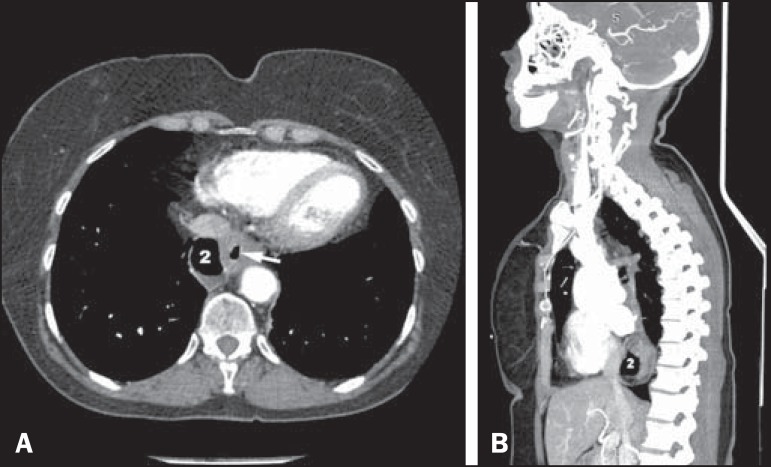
A: Axial CT slice, with a mediastinal window, demonstrating air
